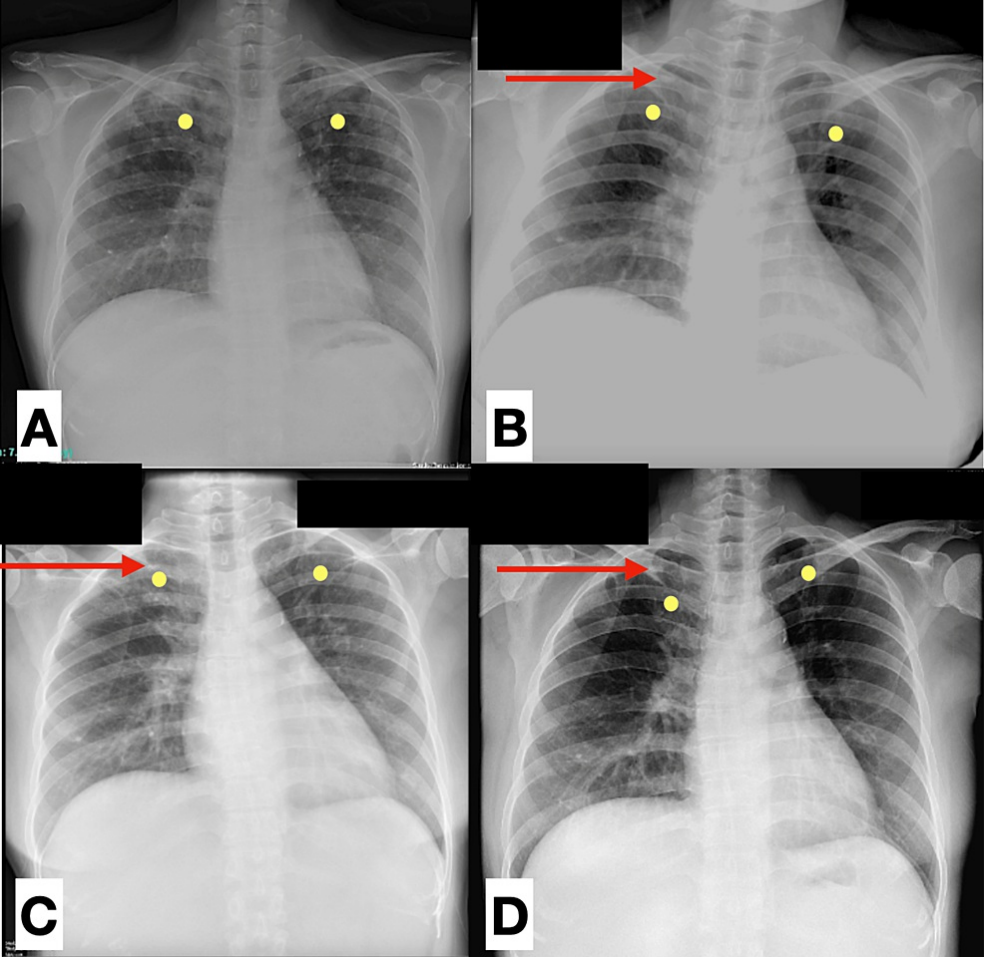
Chest x-rays Chest x-rays were obtained at five years prior (A) , three years prior (B), one year prior (C), and admission (D). The hazy densities (yellow circles) in both upper lungs are noted to be regressing on subsequent chest x-rays with only few remaining densities at the time of admission (D). The small ovoid density on the right upper lung (red arrow) was first seen three years prior (B) and appears stable in subsequent chest x-rays done in (C) and (D), likely relating to a pulmonary tuberculosis with granuloma formation.
